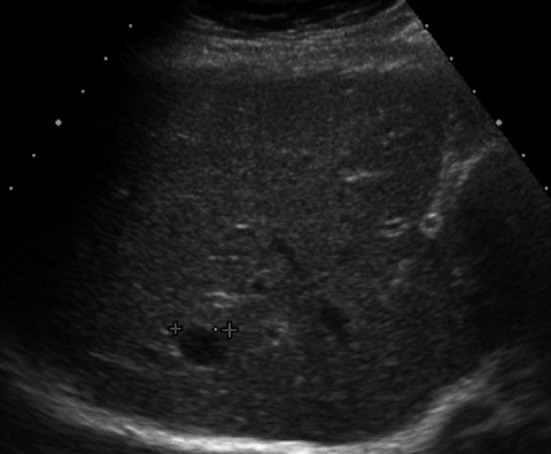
Abdominal ultrasonography showed a hypoechoic lesion, 13.4 mm in diameter, in segment 8 in the liver
